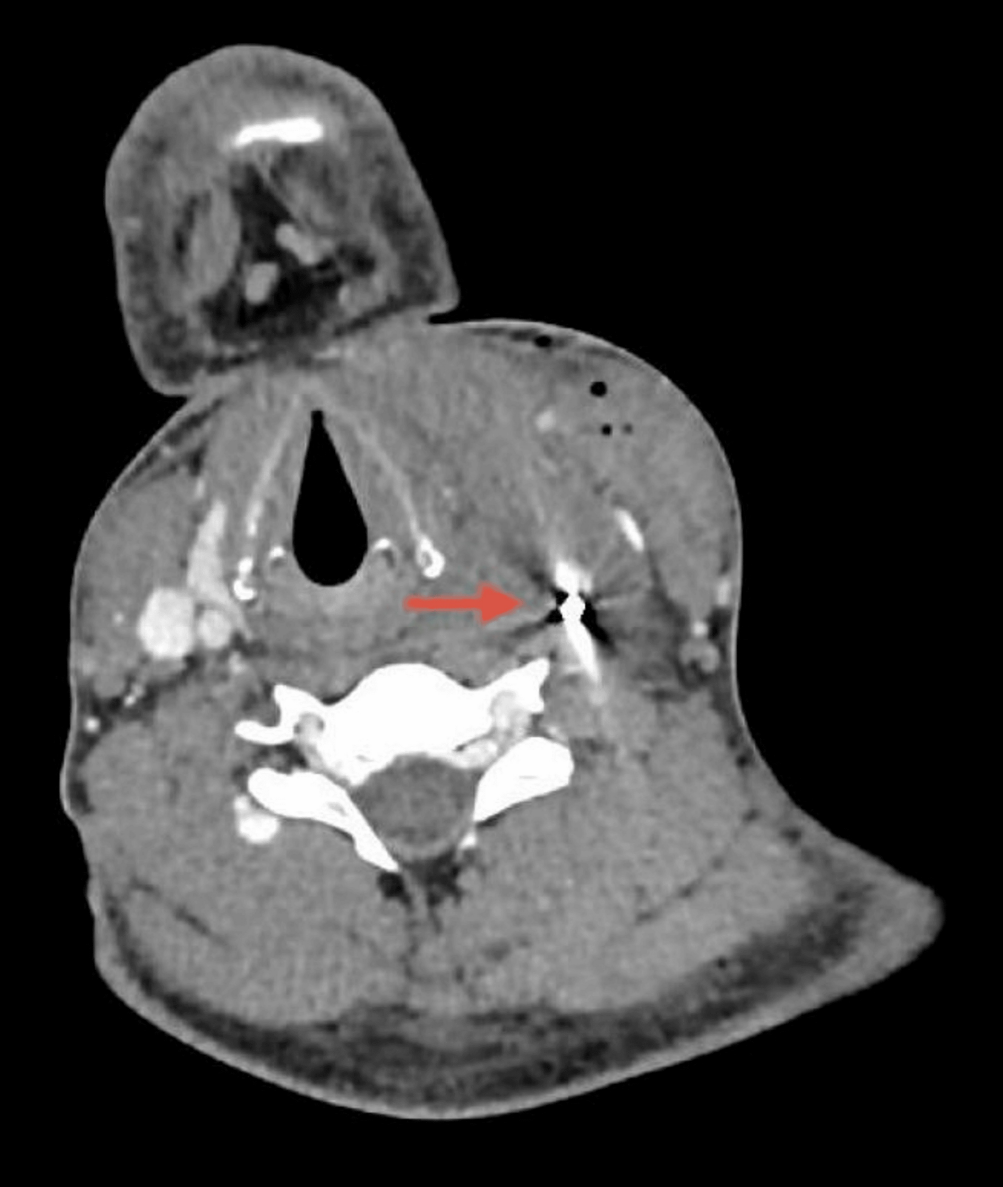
Preoperative CT. Left neck swelling showing air foci associated with shifting of the trachea to the right, suspicious for a muscle tear. A left metallic foreign body with metallic artefact was noted very close to and abutting the common carotid artery.
